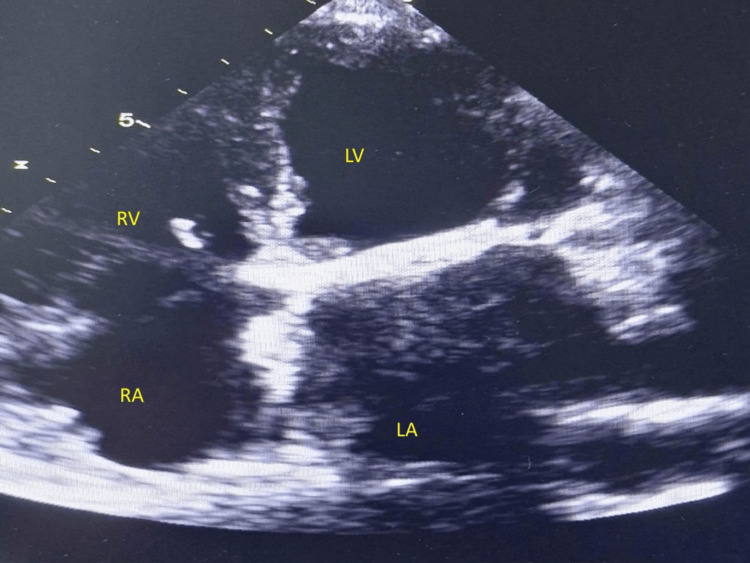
Echocardiography after thrombolysis demonstrating thrombus resolution in four chamber apical view (4C) during first presentation 4C - apical four chamber view, LA - left atrium; LV - left ventricle; RA - right atrium; RV - right ventricle
